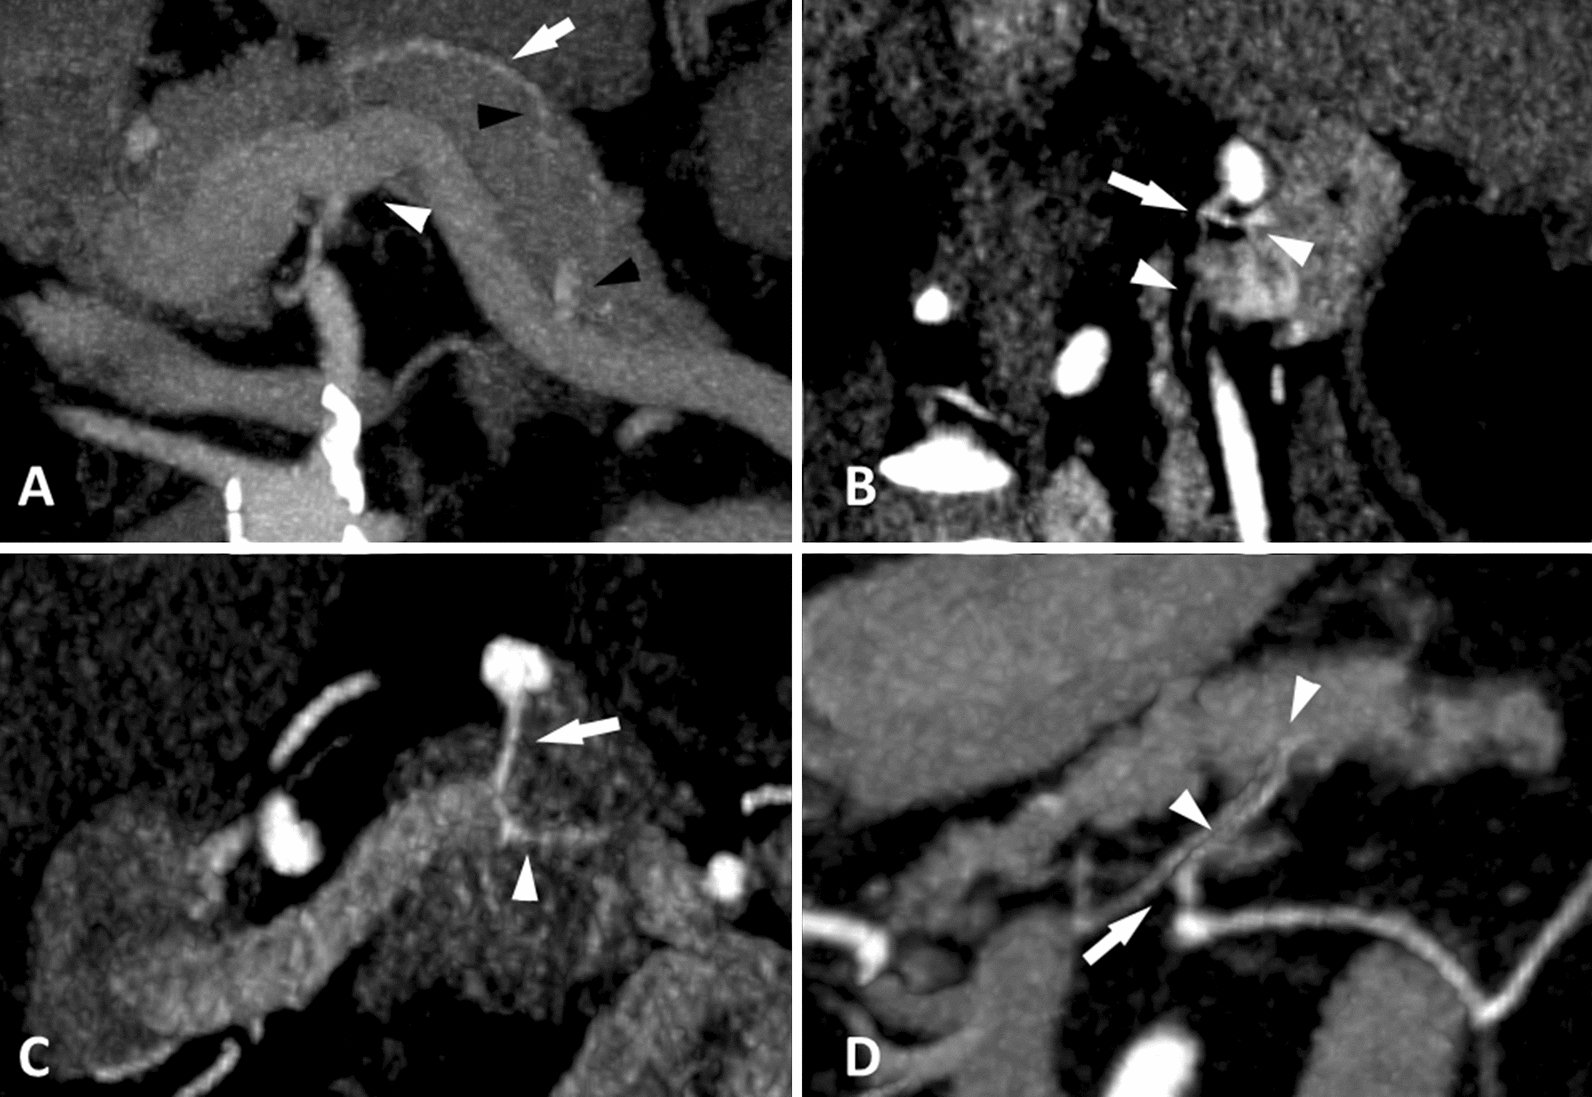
Multi-detector computed tomography after intravenous administration of iodinated contrast material at the arterial phase. a Maximum intensity projection in the transverse plane demonstrates pancreatic transverse (*arrow*) artery communicating with the greater pancreatic artery (*arrowhead*) with first- and second-order vessel division (*black arrowheads*). b Multiplanar oblique reconstruction reveals a dorsal pancreatic artery (*arrow*) with first- and second-order vessel division (*arrowheads*). c Maximum intensity projection with oblique reconstruction demonstrates greater pancreatic artery (*arrow*) with first order vessel division (*arrowhead*). d Maximum intensity projection with oblique reconstruction reveals inferior pancreatic artery (*arrow*) with first- and second order vessel division (*arrowheads*)
